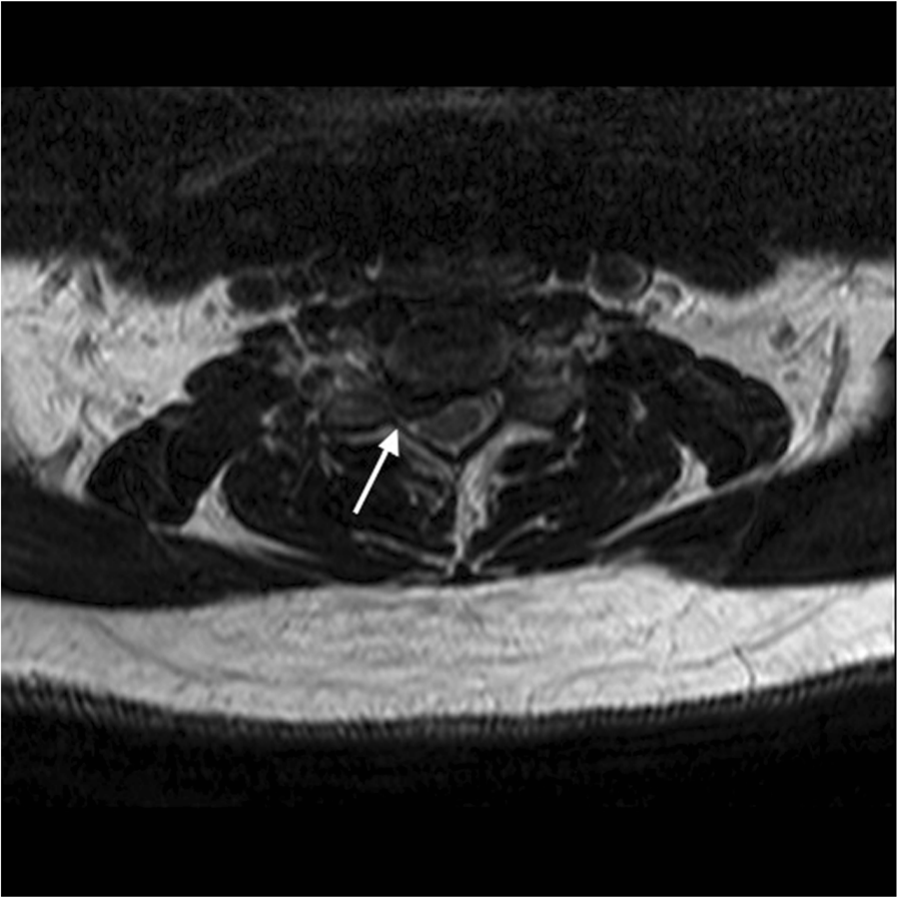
Pre-operative magnetic resonance imaging scan. The arrow on the axial T1-weighted cervical spine image shows the cervical cord posteriorly displaced at the level of the C5 to C6 disc space.
